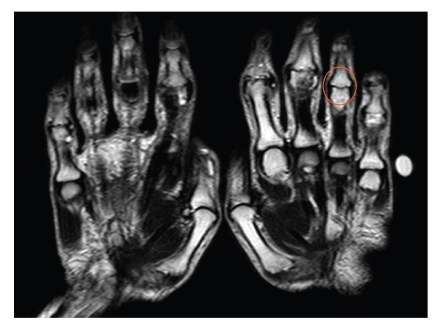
MRI of the proximal interphalangeal joints of both hands confirms sparing of the left ring proximal interphalangeal joint. All other proximal interphalangeal joints show marked synovitis and erosions.
